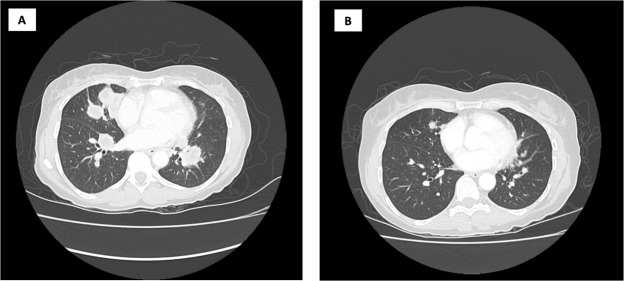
Computed tomography of a patient with undifferentiated pleomorphic sarcoma at cycle 2 (A) and cycle 6 (B) showing a partial response.
